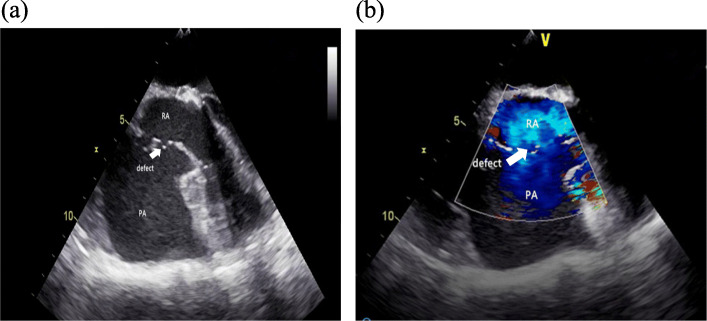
Intraoperative TEE. Intraoperative transoesophageal echocardiography shows right atrium rupture with pseudoaneurysm formation. PA: pseudoaneurysm; RA: right atrium
